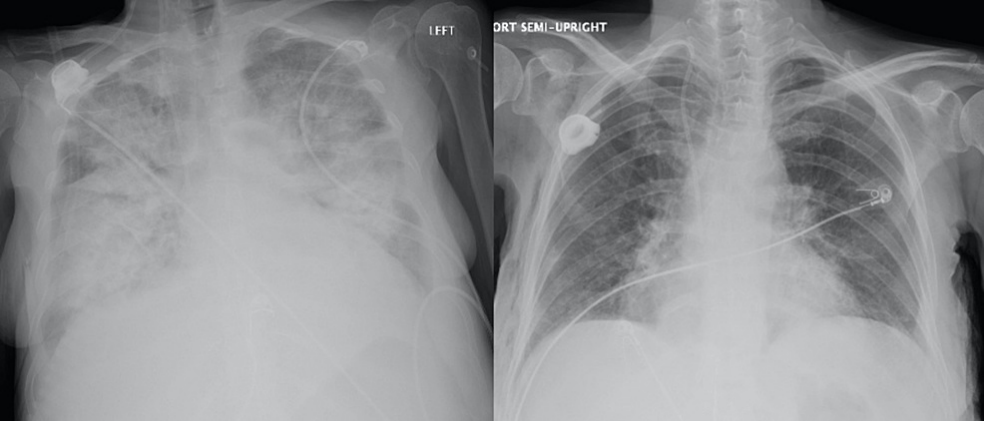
Chest X-rays Left: day of admission with severe bilateral pulmonary disease; Right: Day of discharge with the resolution of the disease
